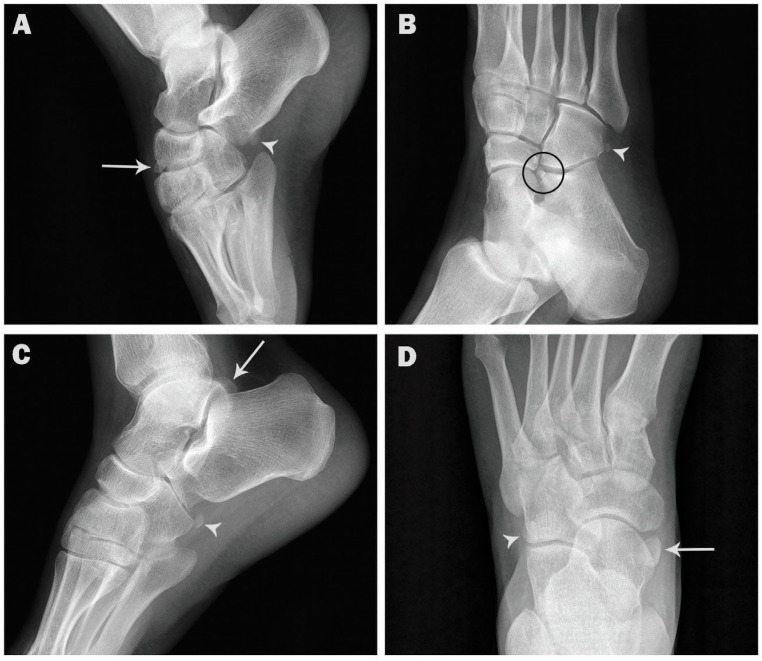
Coexistence of os peroneum and other ossicles in the same foot. (A). Lateral radiograph showing os peroneum (arrowhead) and os infranaviculare (arrow) in a 31-year-old female. (B). Oblique radiograph showing os peroneum (arrowhead) and os calcaneus secundarius (circle) in a 52-year-old female. (C). Lateral radiograph showing os peroneum (arrowhead) and os trigonum (arrow) in a 48-year-old male. (D). AP radiograph showing os peroneum (arrowhead) and accessory navicular (arrow) in a 55-year-old male.
